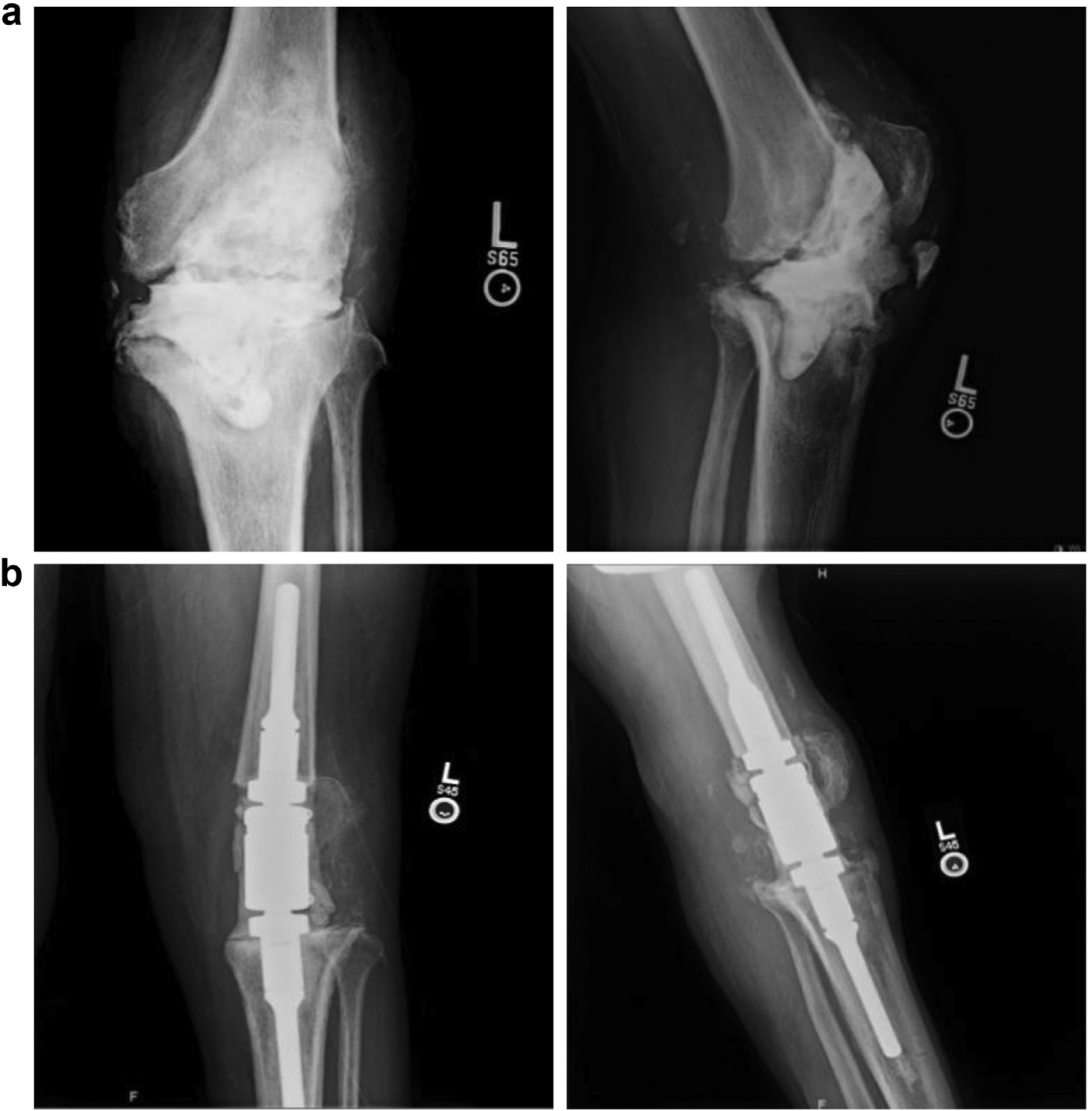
Radiographs of a 67-year-old male with (a) persistent *Pseudomonas aeruginosa* periprosthetic joint infection despite explantation and articulating spacer placement. (b) The Patient underwent knee fusion with the modular endofusion system and has no evidence of infection at the latest follow-up.
